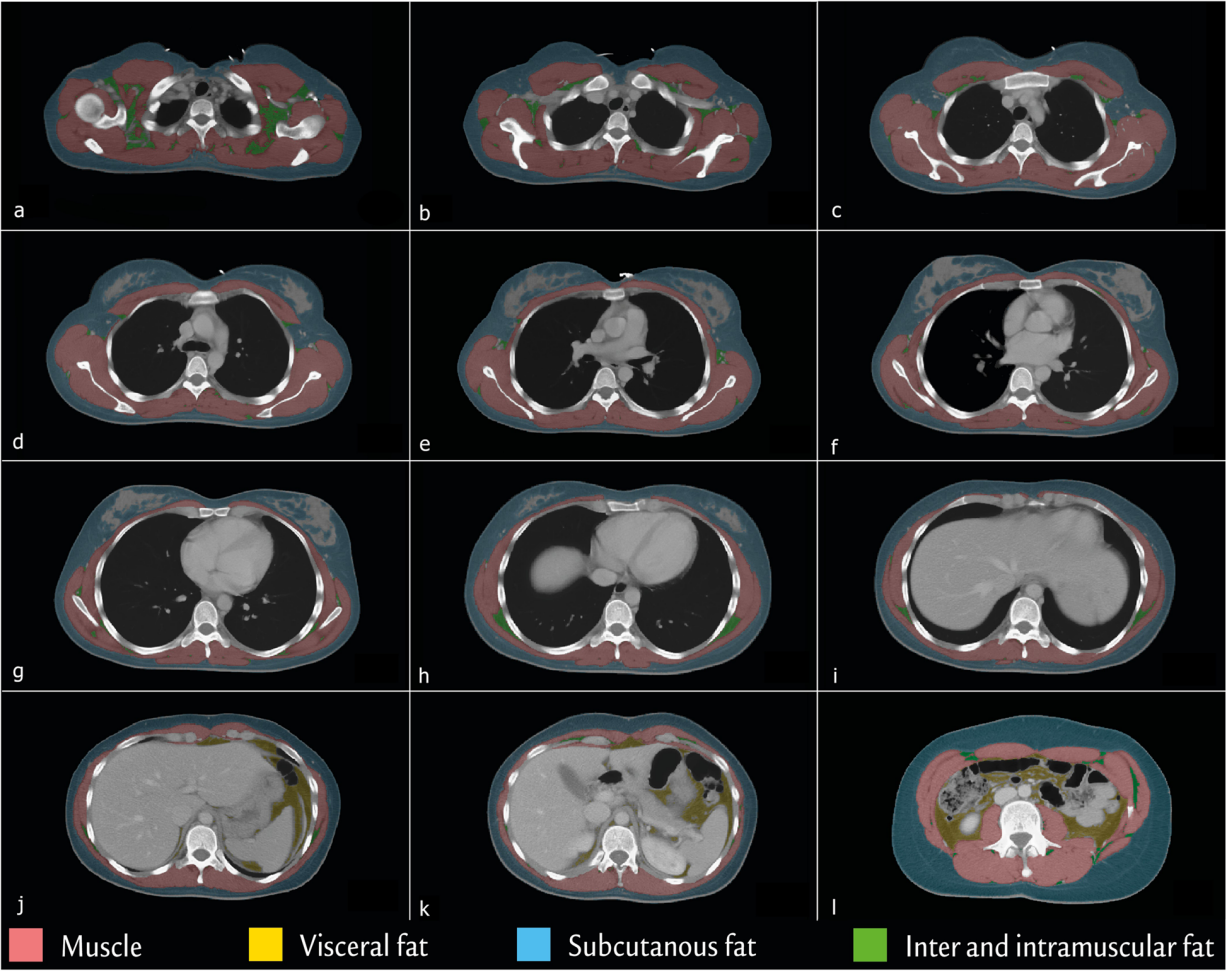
Example of segmentation of the axial images from a contrast-enhanced thoracic and abdominal computed tomography scan on a 27-year-old female. Thoracic level 2 (a), thoracic level 3 (b), thoracic level 4 (c), thoracic level 5 (d), thoracic level 6 (e), thoracic level 7 (f), thoracic level 8 (g), thoracic level 9 (h), thoracic level 10 (i), thoracic level 11 (j), thoracic level 12 (k), and lumbar level 3 (l)
